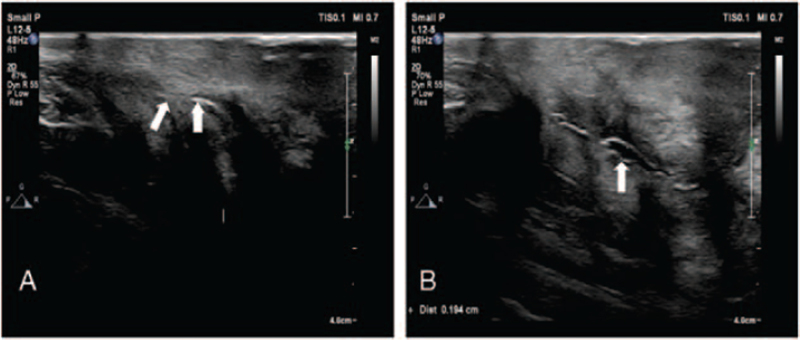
Postoperative ultrasonography at 45 days follow-up. A. The high frequency probe shows almost healed wound (arrows). B. The visualization of urethra in ultrasound scan suggested the presence of a small effusion(arrow).
